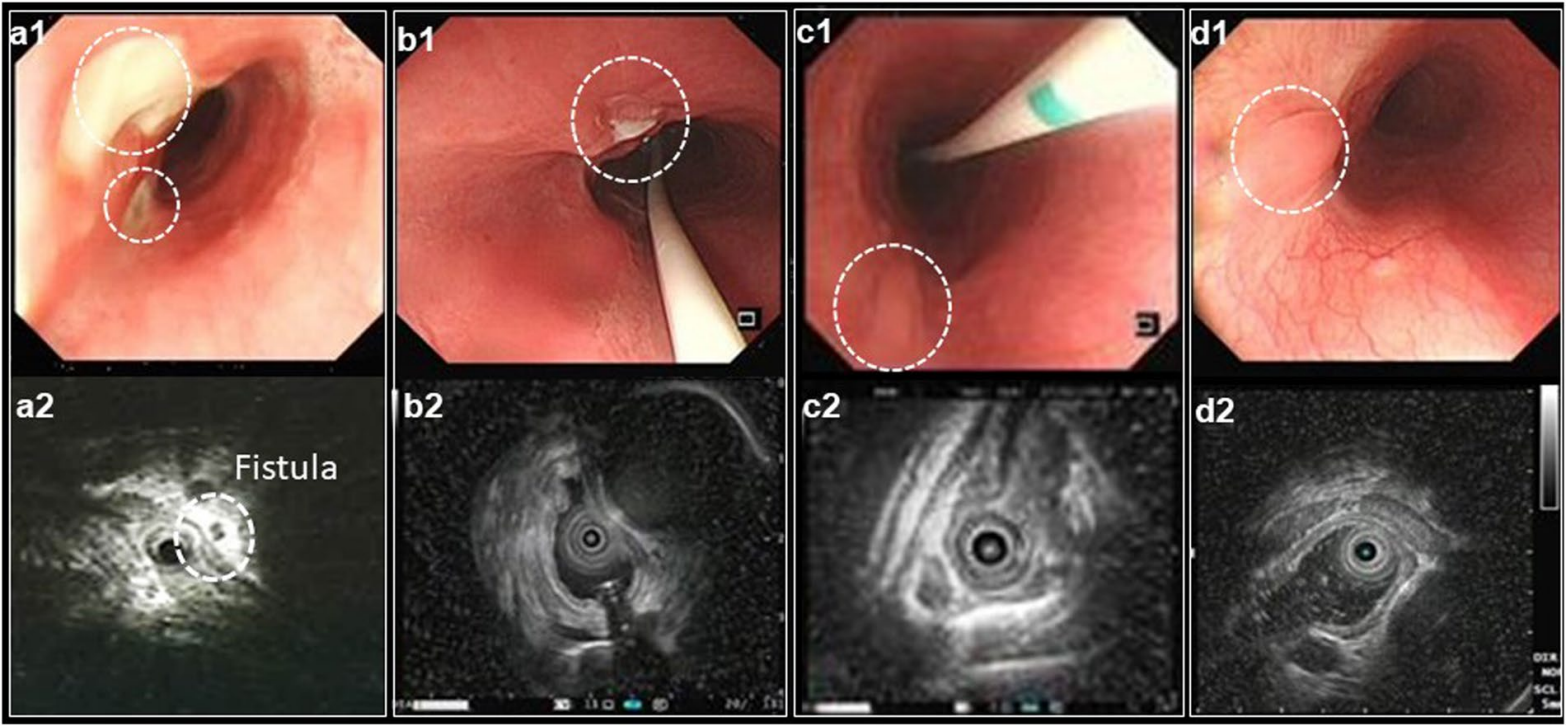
Evolution of the esophagopericardial fistula post ablation. The gastroscopy demonstrated the progression of esophagopericardial fistula 7 days (a1) 14 days (b1), 21 days (c1) and 30 days (d1) after RF ablation. Ultrasonography revealed dense echo with air, which indicated local fistula formation (a2, white dot circle) and recovery of esophageal tissue layer (b2,c2,d2).
